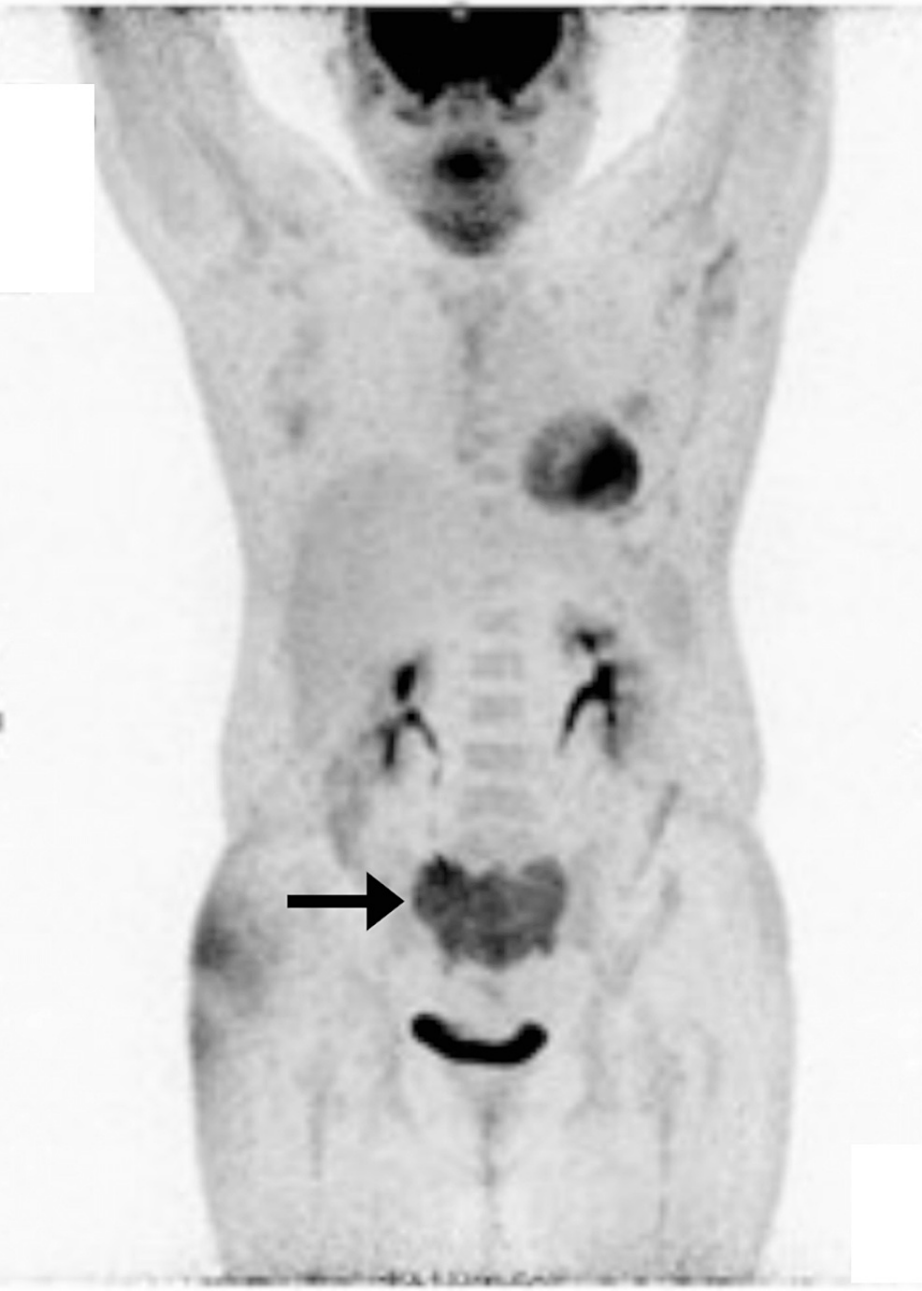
Fluorodeoxyglucose whole-body positron emission tomography/computed tomography. Increased fluorodeoxyglucose uptake is evident within the sacrum (arrow), with a standardized uptake value of 9.82.
